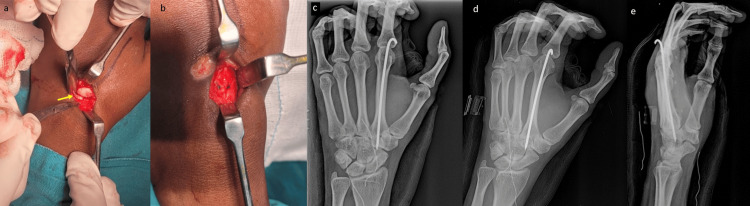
Intra-operative pictures and postoperative X-rays (a) Intra-operative picture showing the base of the second metacarpal with the interposed extensor tendon (arrow) between the base of second metacarpal and the trapezoid, (b) sutured dorsal capsule. Postoperative X-rays showing the reduced second carpometacarpal joint transfixed with a K-wire; (c) anteroposterior, (d) oblique and (e) lateral views
